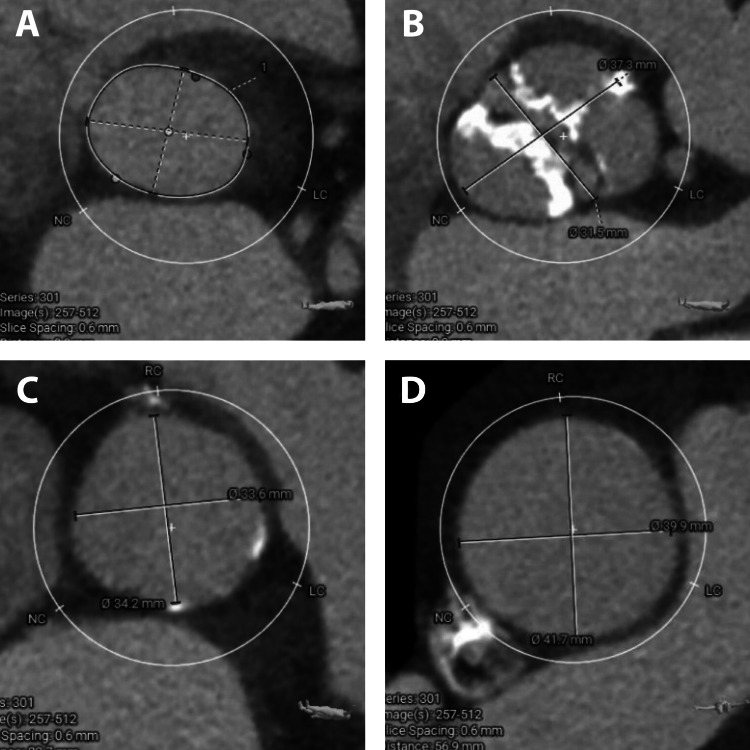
Pre-procedural transcatheter aortic valve replacement computed tomography angiogram measurements (2) A - Aortic annulus measurements, B - sinus of Valsalva measurements, C - sinotubular junction measurements, D - proximal ascending aorta measurements
